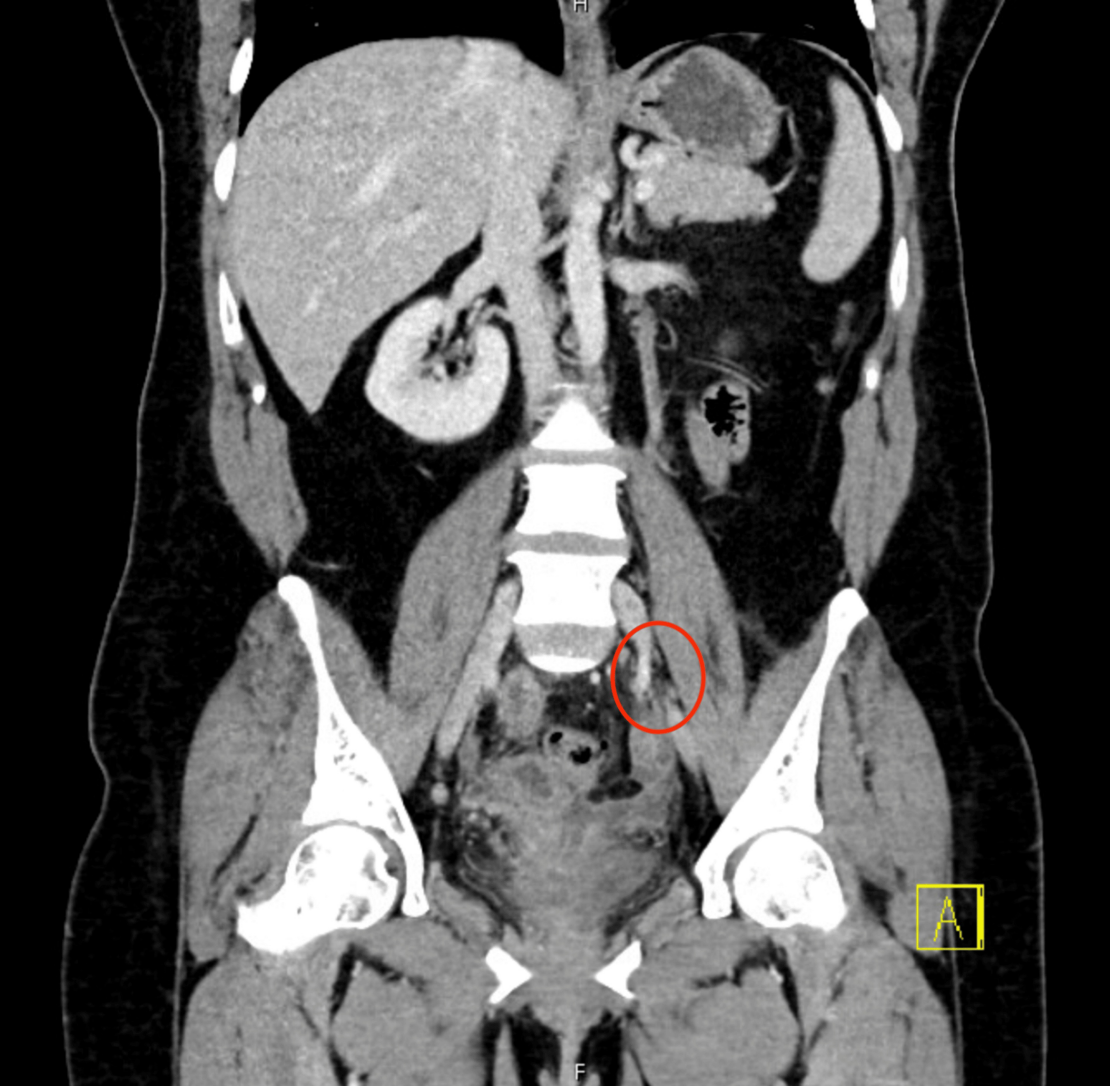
Coronal CT of the abdomen and pelvis demonstrating left gonadal vein filling defect
